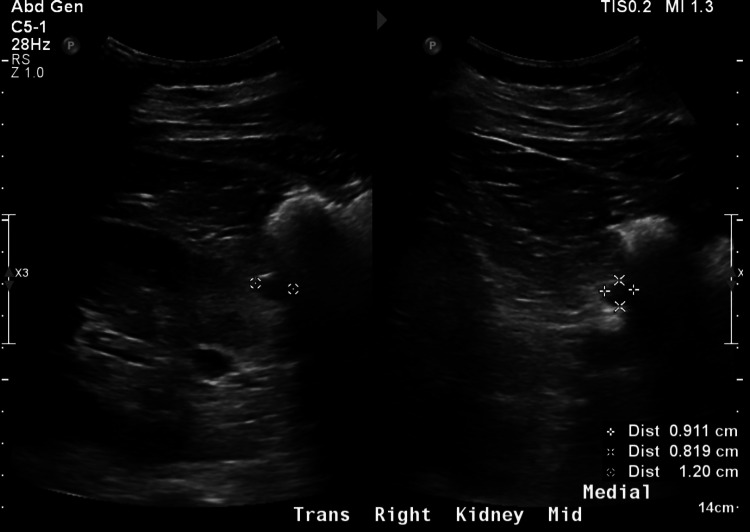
Kidney ultrasound reveals a simple-appearing cyst in the midpole of the right kidney, measuring 1.2 cm
